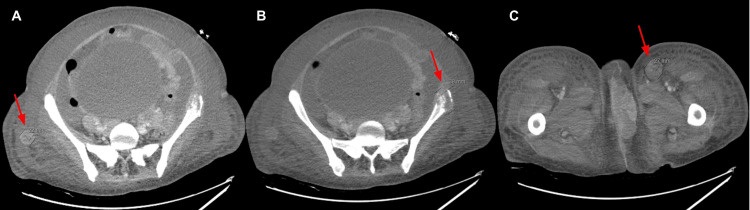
CT of the abdomen/pelvis showing multiple soft tissue masses in the right gluteal fat with the largest measuring 2.2 cm in axial view indicated by the red arrow (A), expansive lytic lesion in the left iliac measuring at least 3.9 cm in axial view indicated by the red arrow (B), and bulky left inguinal adenopathy with one node measuring at least 2.7 cm in axial view indicated by the red arrow (C). CT: computed tomography
